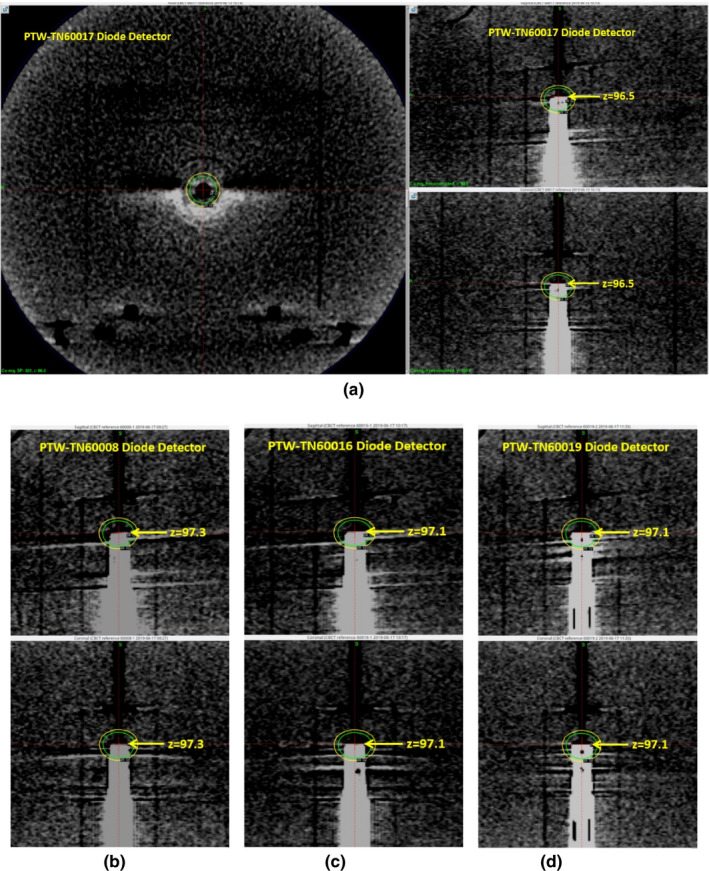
Axial (left), sagittal (right upper), and coronal (right left) views of (a) the CBCT scan of the PTW‐TN60017 diode detector. The yellow line in each panel marks the most superior part of the detector that can be identified from the scan. Also shown in (b)–(d) are the sagittal (upper) and coronal (lower) views of the other three diode detector.
